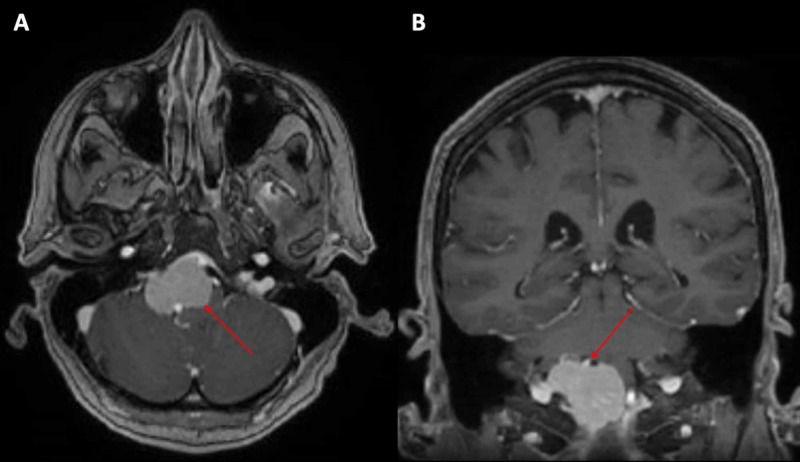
(A) Axial and (B) coronal views of magnetic resonance imaging of the brain at the time of diagnosis revealing right inferior clival meningioma.
